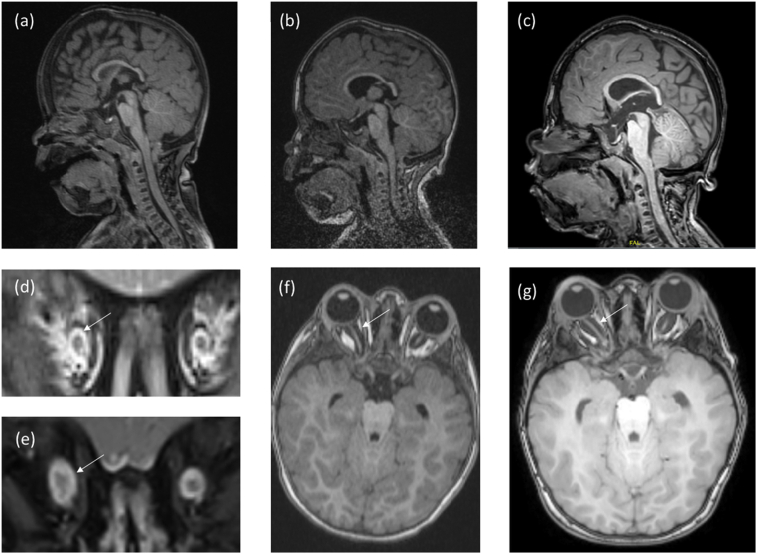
MRI of the brain at 1.5 years (a,d), 3.8 years (b,f) and 5.3 years of age (c,e,g). Sagittal T1 images. (a,b,c) show a progressive enlargement of the sella and approximation of the clivus and the pons with increasing flexure of the lower brainstem. The cerebellar tonsils are deep, but do not protrude into the foramen magnum. The subarachnoidal space around the optic nerve is progressively enlarged (Arrows in d,e,f,g) without papilledema or signs of increased intracranial pressure. Myelination shows no alteration in T1 (f,g) or T2 weighted images (data not shown).
